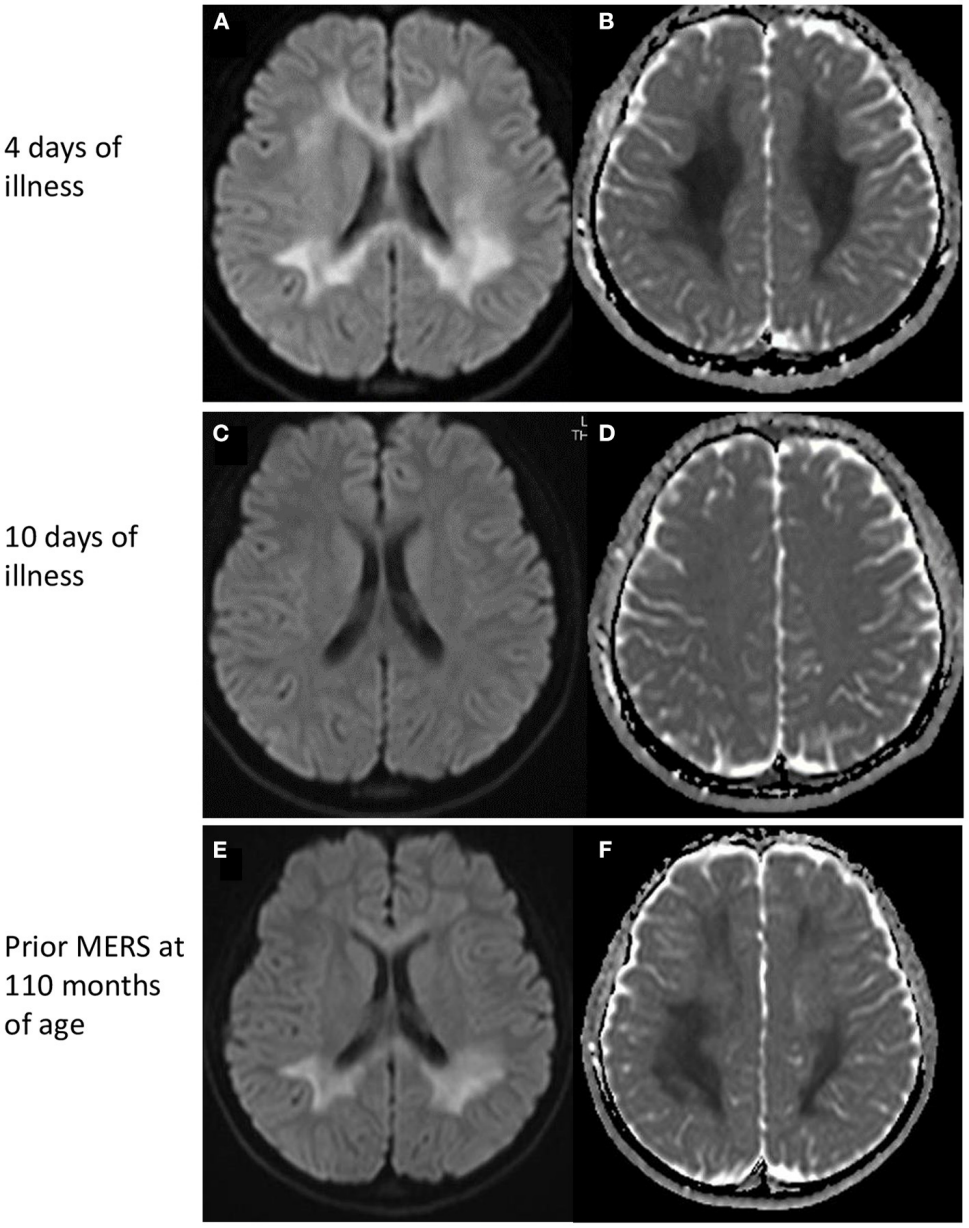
MRI findings. (Top) MRI on day 4 of illness. Restricted water diffusion was observed in the corpus callosum and deep white matter. (A) Diffusion-weighted images, and (B) apparent diffusion coefficients map. (Middle) MRI on day 10 of illness. No MRI abnormalities were seen. (C) Diffusion-weighted images, and (D) apparent diffusion coefficients map. (Bottom) MRI of a prior MERS episode at 110 months of age. A similar pattern of restricted water diffusion is evident in the corpus callosum and deep white matter. (E) Diffusion-weighted images, and (F) apparent diffusion coefficients map. MERS, Mild encephalitis/encephalopathy with a reversible splenial lesion.
